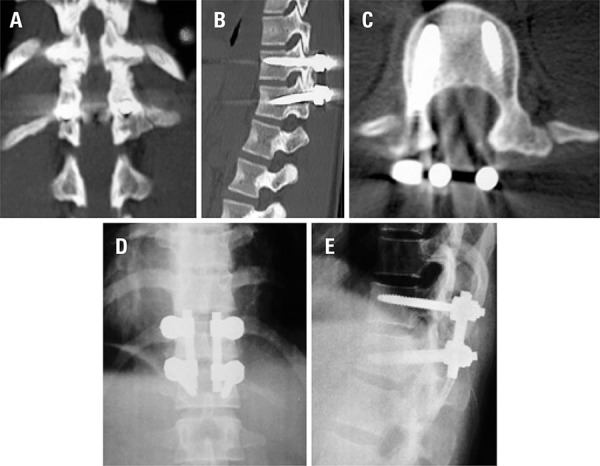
(A) coronal computed tomography scan showing complete T11-T12 fusion; (B) sagittal computed tomography scan showing complete T11-T12 fusion; (C) axial postoperative computed tomography scan; (D) postoperative anteroposterior X-ray showing proper coronal spine alignment; (E) postoperative lateral X-ray showing proper sagittal spine alignment
